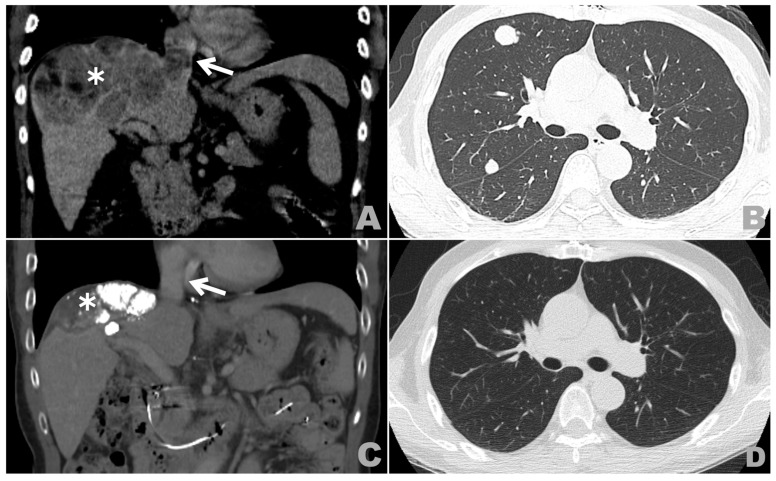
Key imaging studies with different treatment modalities: (A) contrast-enhanced CT of upper abdomen with coronal reconstruction at initial diagnosis shows a huge liver tumor (white asterisk, *) with tumor thrombi (white arrow) in inferior vena cava (IVC) and right atrium (RA). (B) Chest CT after hepatic artery infusion chemotherapy (HAIC) and drug-eluting bead transarterial chemoembolization (DEB-TACE) shows progressive metastatic lung nodules. (C) Follow-up contrast-enhanced CT of upper abdomen with coronal reconstruction after multimodal treatment shows tumor shrinkage with cystic necrosis and lipiodol retention (white asterisk, *) and resolution of previous RA and IVC tumor thrombi (white arrow). (D) Follow-up chest CT after 8 cycles of nivolumab shows clear bilateral lung fields without metastatic lung nodules.
